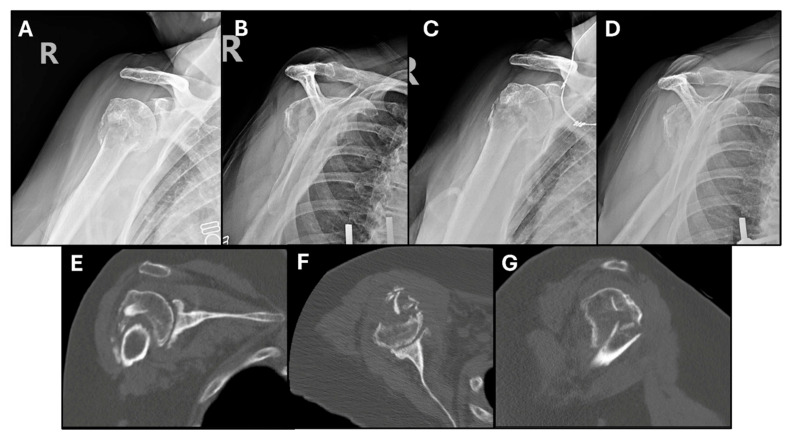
Selected radiographs and CT scan cuts from our patient’s right (R) shoulder. (A,B) Initial injury radiographs of the right shoulder demonstrating surgical neck fracture. (C,D) Radiographs obtained after 6 weeks of nonoperative management, showing interval varus displacement and minimal callus formation. (E–G) Coronal, axial and sagittal CT cuts at 10 weeks post-injury depicting no significant bridging callus at the fracture site. Underlying glenohumeral arthritis is also observed.
